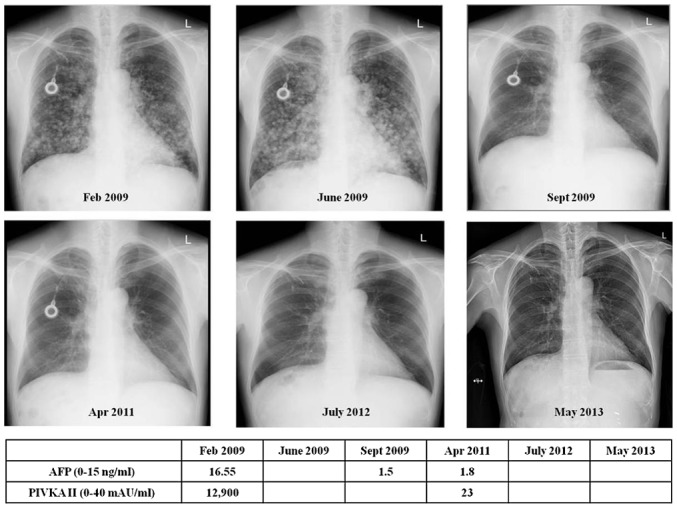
Chest radiograph revealed no metastatic lesions in either lung field from September, 2009. Levels of AFP and PIVKA II were within the normal range. AFP, α-fetoprotein; PIVKA II, protein induced by vitamin K absence or antagonist II.
